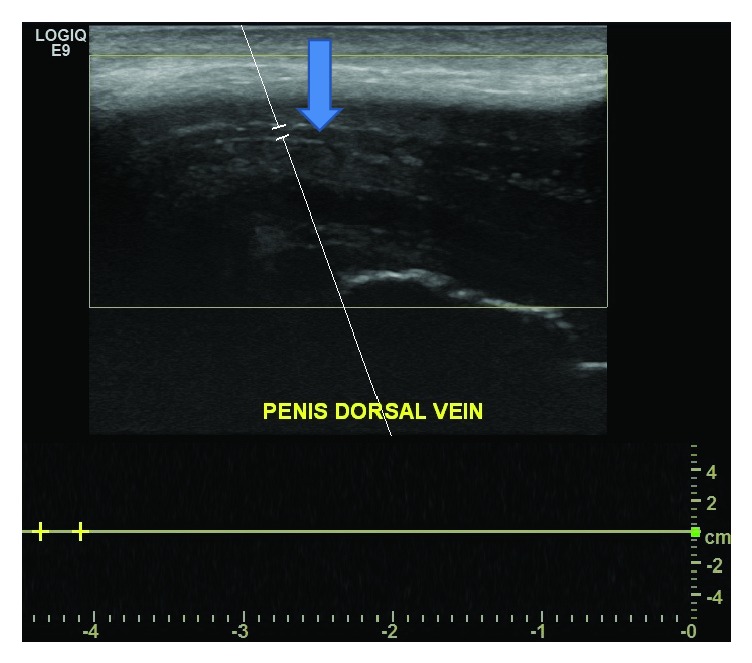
Penile ultrasound with the arrow directed towards Doppler noting absence of flow in dorsal vein.
